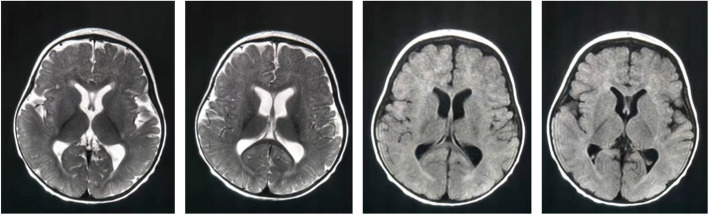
Magnetic resonance imaging scans when the girl was 10 months old show abnormal signals in the posterior horn and trigonometry region of the lateral ventricle; the lateral ventricle and the third ventricle were slightly enlarged
